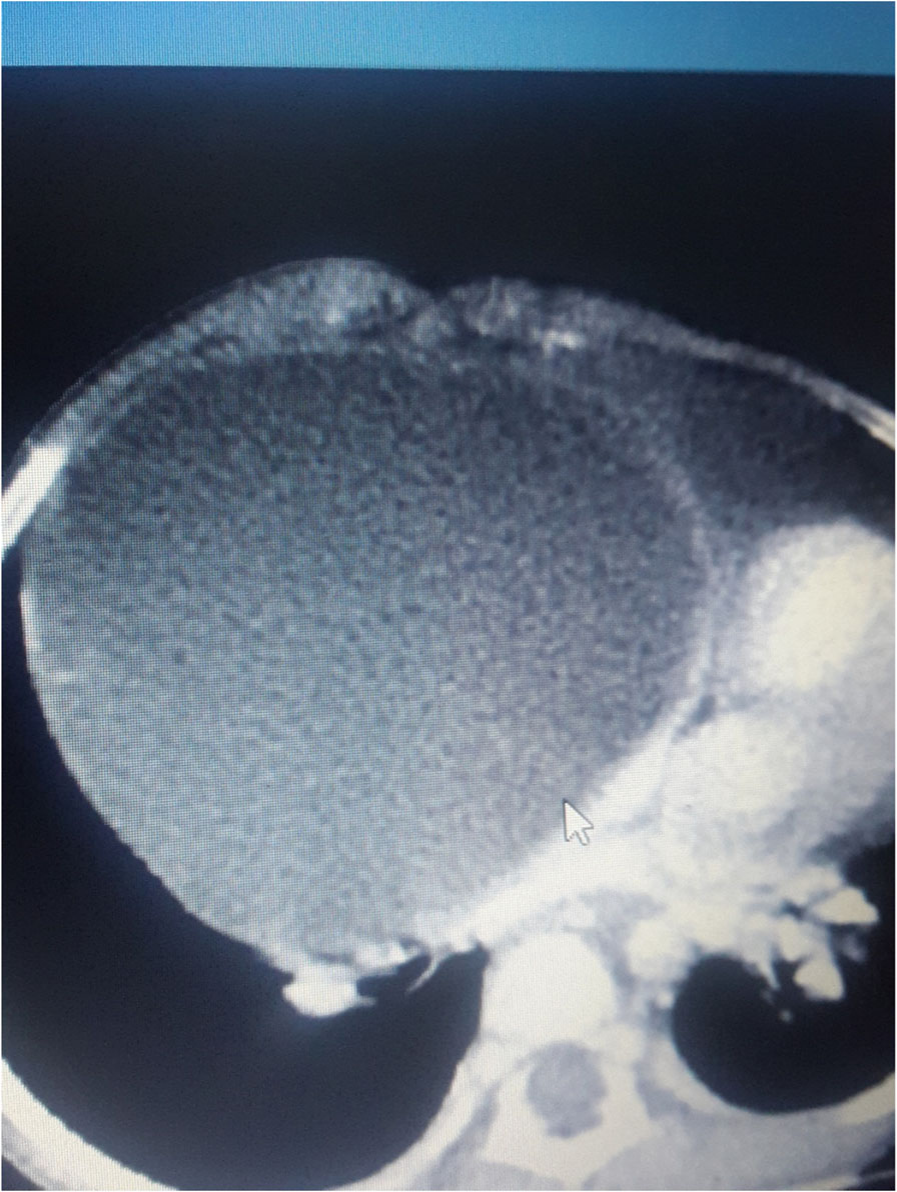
Contrast CT scan showing the cyst(white arrow)
